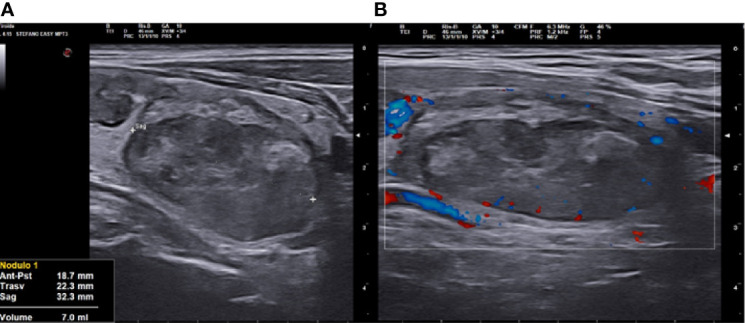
Right lobe nodule, longitudinal ultrasound (US) scan at 3 months follow up after vascular radiofrequency ablation (vRFA). (A) The nodule shrunk from 18.5 to 7 ml in 3 months, strongly hypoechoic, solid. (B) at Color Doppler (CD) evaluation, absence of peri- and intranodular vascular signal.
